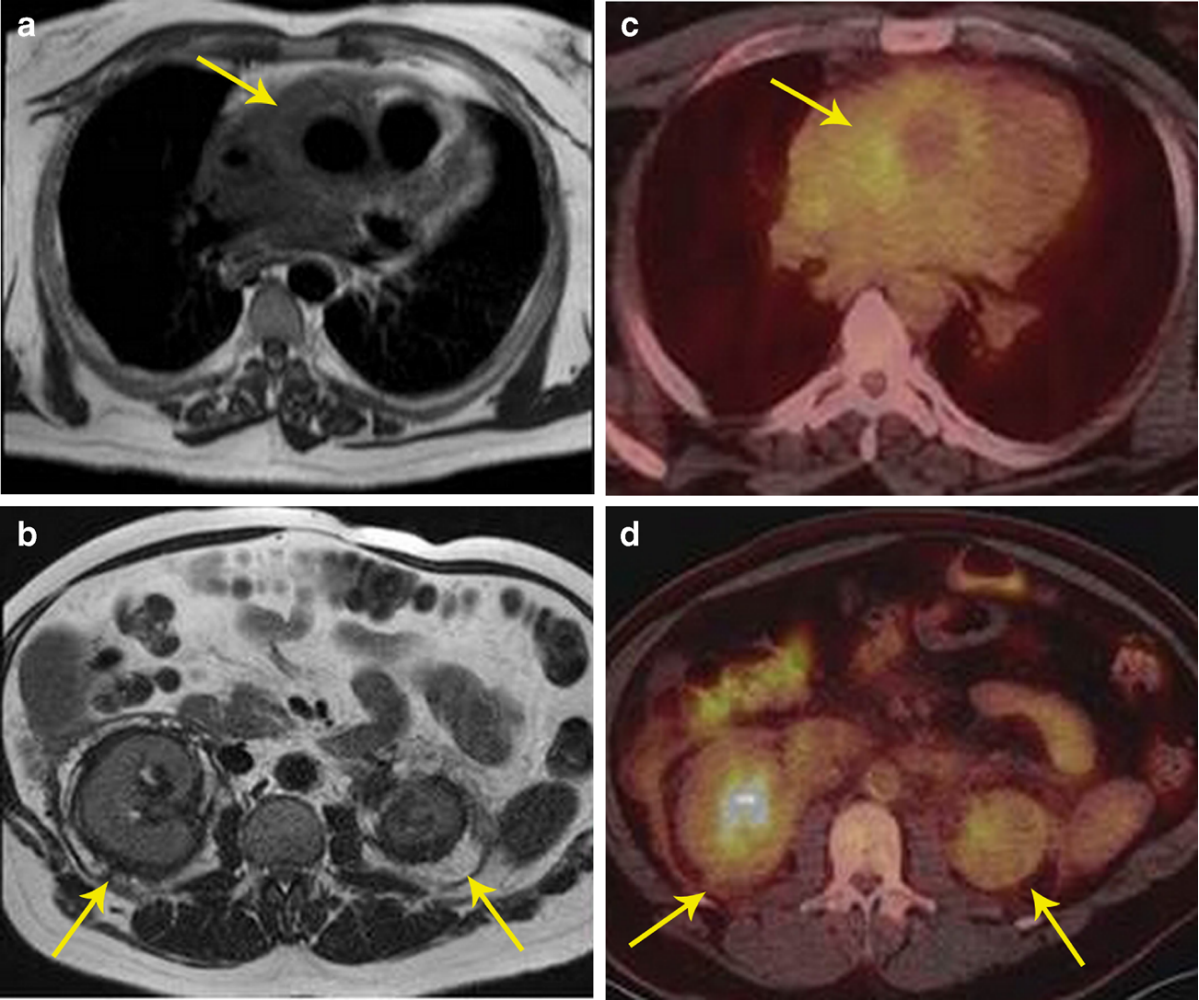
Inflammatory disease. a 46-year-old male with history notable for hypertension, bilateral orbital xanthogranulomas, and chronic low-grade fevers. As part of work-up, the patient had an aortic MRI that demonstrated diffuse confluent soft tissue within the mediastinum (arrow). b There were additional soft tissue changes as well as stranding surrounding both kidneys (arrows). c FDG PET/CT showed only minimal FDG avidity in the paraaortic soft tissue. d FDG-PET/CT also showed only minimally increased uptake around the kidneys. Bone scan (not shown here) was notable for diffusely increased uptake along long bones. Given these imaging and clinical findings, chronic inflammatory process with suggestion of mild activity at time of imaging was suspected; laboratory testing of orbital biopsy (CD68, CD163 positive, CD1a negative) led to a diagnosis of Erdheim-Chester disease
